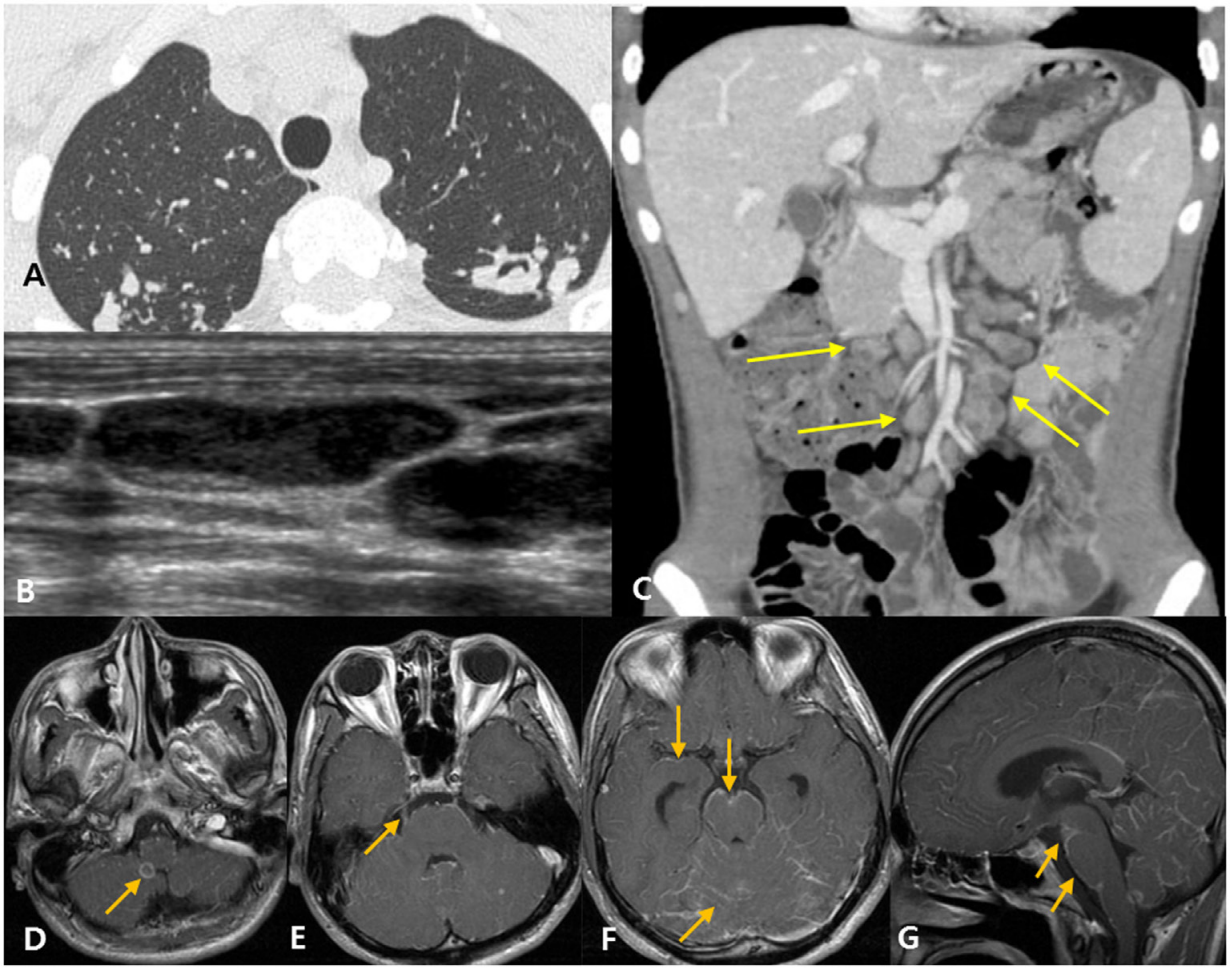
Disseminated tuberculosis case in a 22-year-old man with headache and diplopia. (A) The patient was diagnosed with active pulmonary tuberculosis based on chest computed tomography (CT). (B) Neck ultrasound shows eccentric necrosis in the enlarged lymph nodes of the neck, suggestive of tuberculosis lymphadenitis. (C) Abdominopelvic CT shows mesenteric lymphadenopathy (yellow arrows) and retroperitoneal lymphadenopathy (not shown), suggestive of tuberculosis lymphadenitis. (D–G) Post-contrast T1-weighted magnetic resonance images show rim enhancing tuberculoma [(D), arrow], thick, irregular enhancement of the right trigeminal nerve [(E), arrow], and linear leptomeningeal enhancement along the cerebral sulci, cerebellar folia, and the surface of the brain stem [(F,G), arrows].
